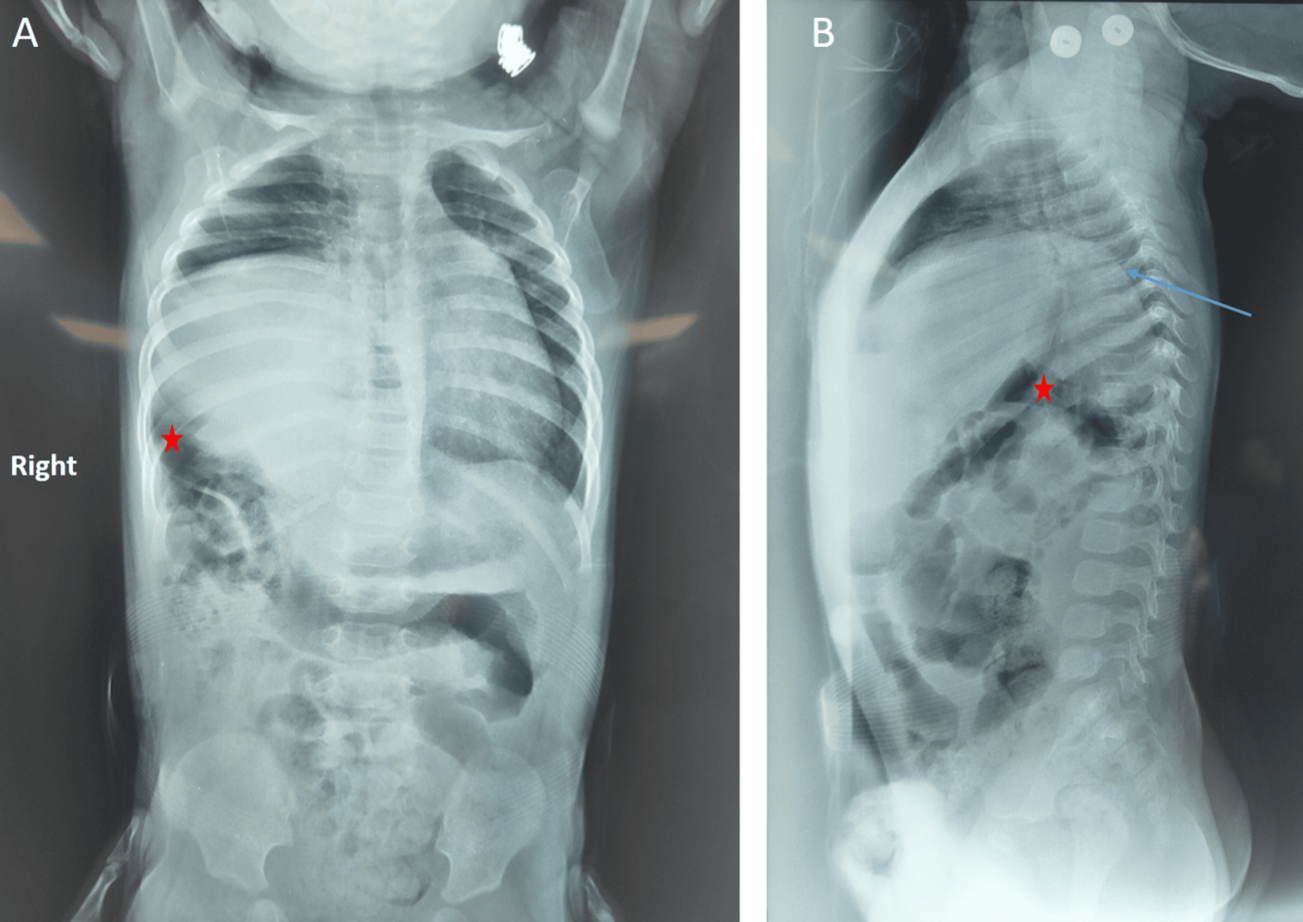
Frontal (A) and lateral (B) chest radiographs of the patient (A) The frontal view shows right-sided lucencies (red star) with mediastinal shift, initially suggestive of a congenital diaphragmatic hernia. (B) The lateral view reveals elevation of the right hemidiaphragm (blue arrow) with intrathoracic bowel loops (red star), consistent with congenital diaphragmatic eventration.
